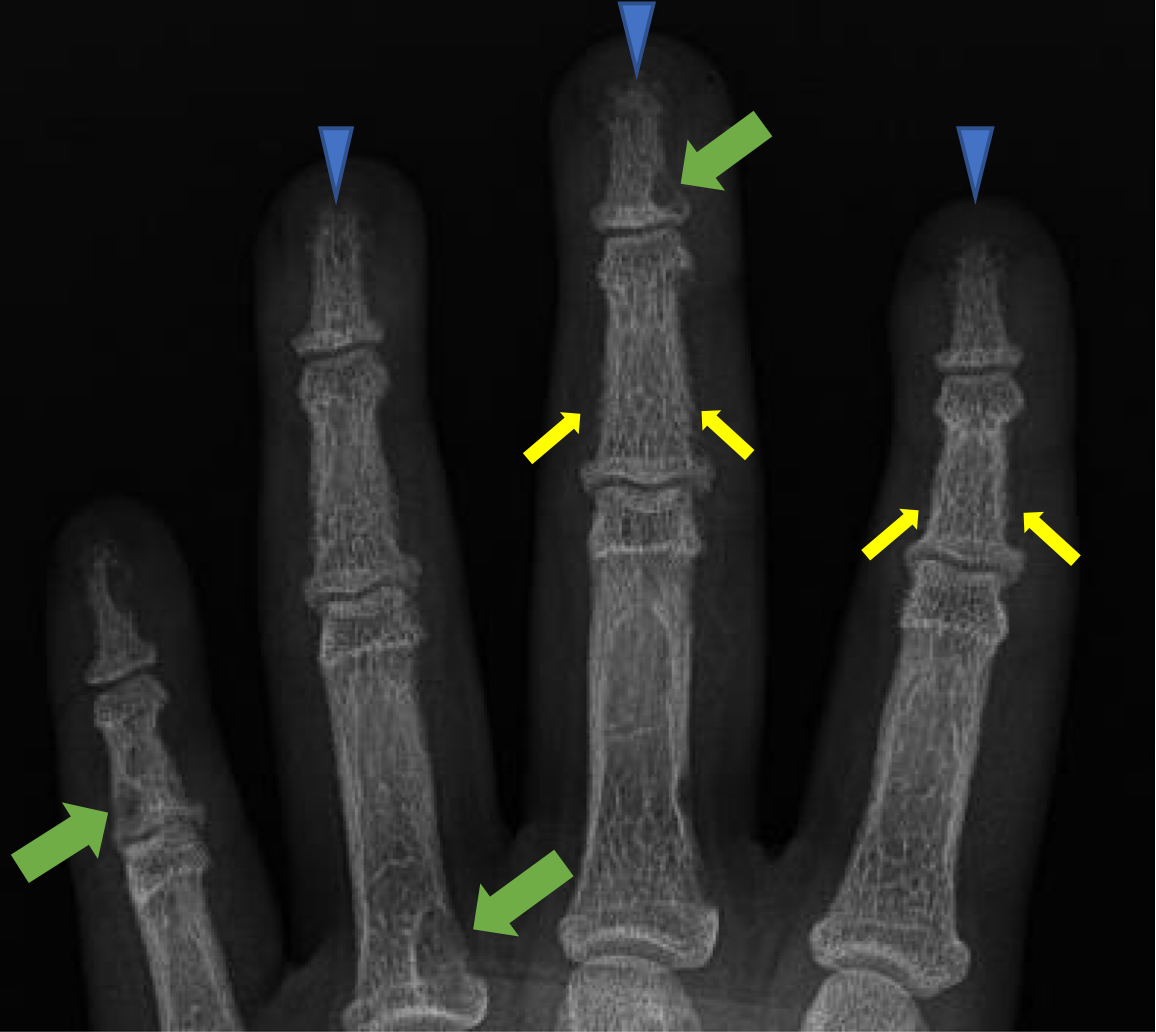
Frontal radiograph of the left hand in a 14 year old male patient with hyperparathyroidism demonstrates acro-osteolysis (arrowheads), subperiosteal resorption at the middle phalanges (small arrows), brown tumors (large arrows) and diffuse osteopenia
